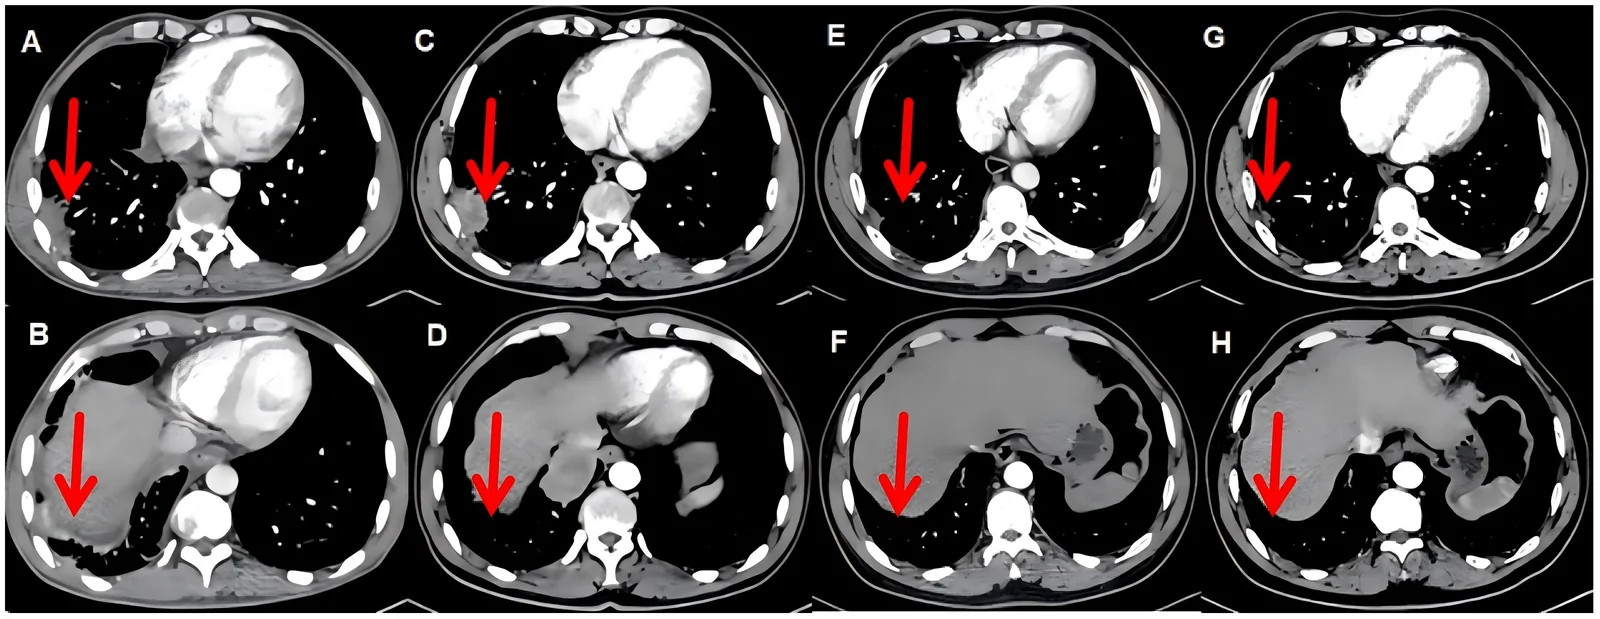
Serial chest CT scans during the disease course and therapeutic response. (A, B) One month after oral crizotinib (Partial Response). (C, D) After 6 months of crizotinib treatment (Progressive Disease). (E, F) After 10 months of lorlatinib treatment (Partial Response). (G, H) 15 months after entrectinib treatment (Partial Response).
